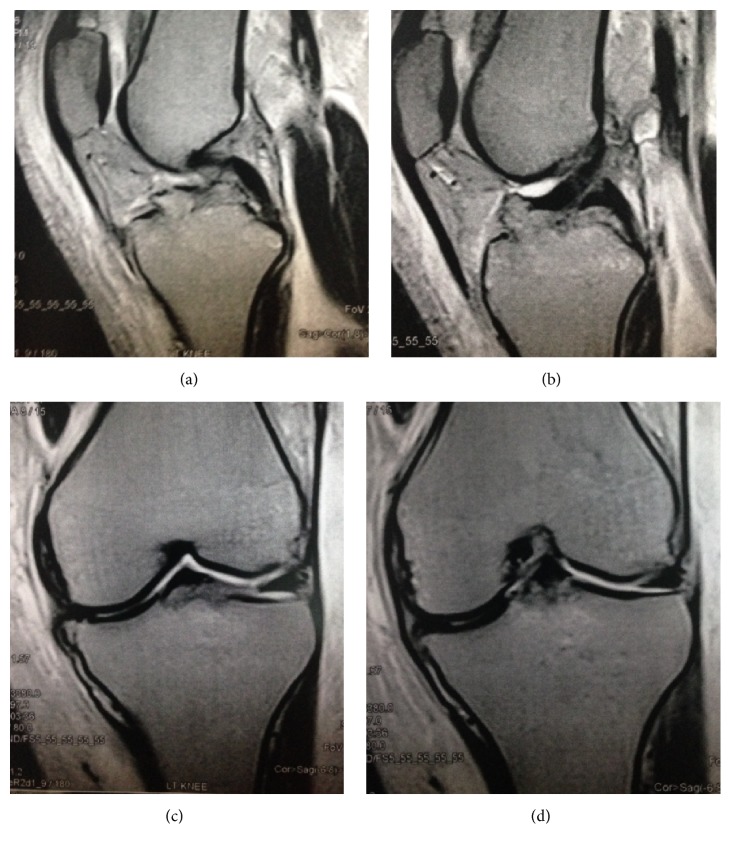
Magnetic resonance imaging showing intact PCL (a), avulsion of the tibial attachment of the ACL (b), reverse Segond fracture (c), and intact superficial MCL (d).
